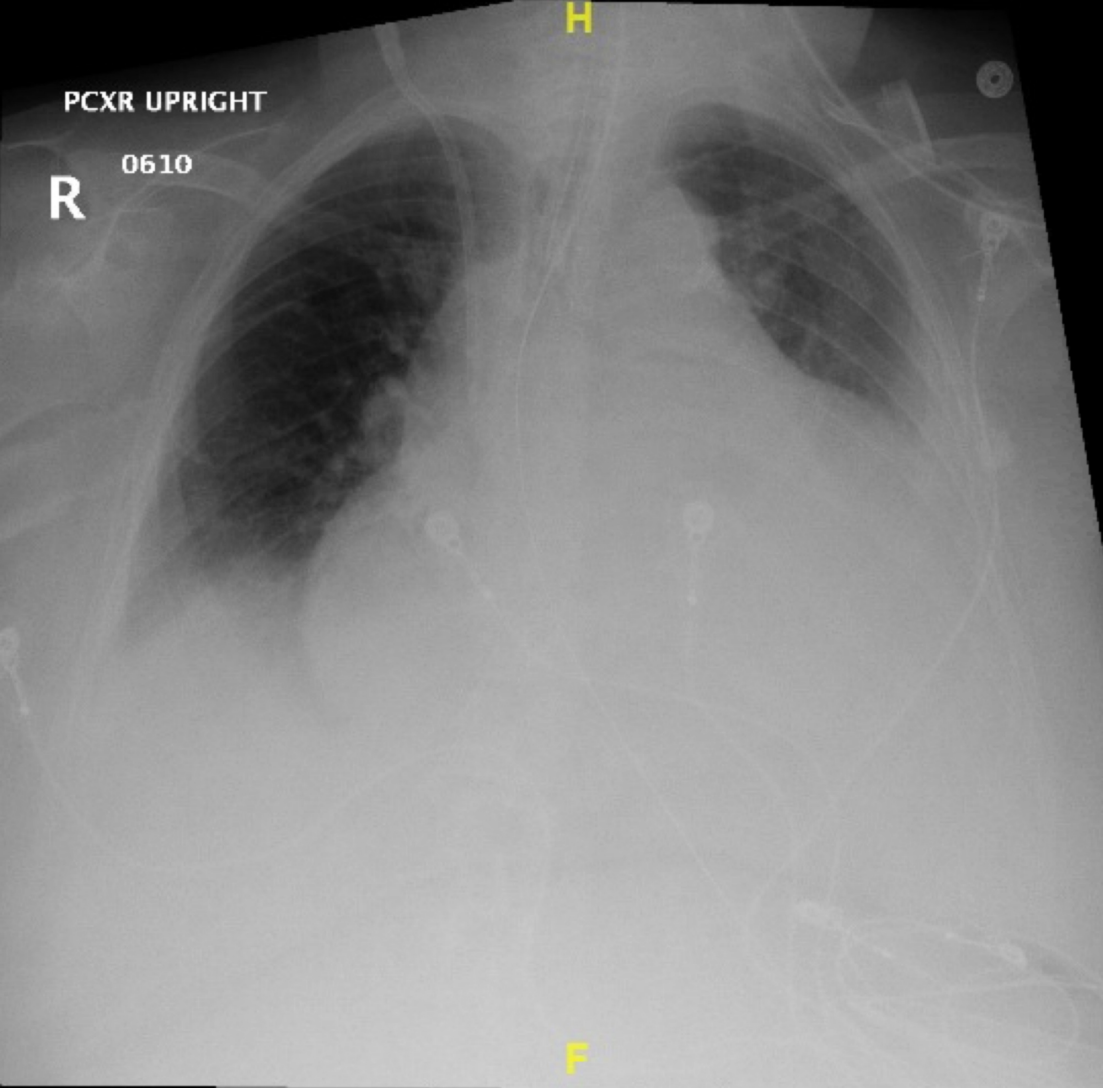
CXR showing left-sided pleural effusion with a water bottle sign concerning for pericardial effusion. CXR, chest X-ray
